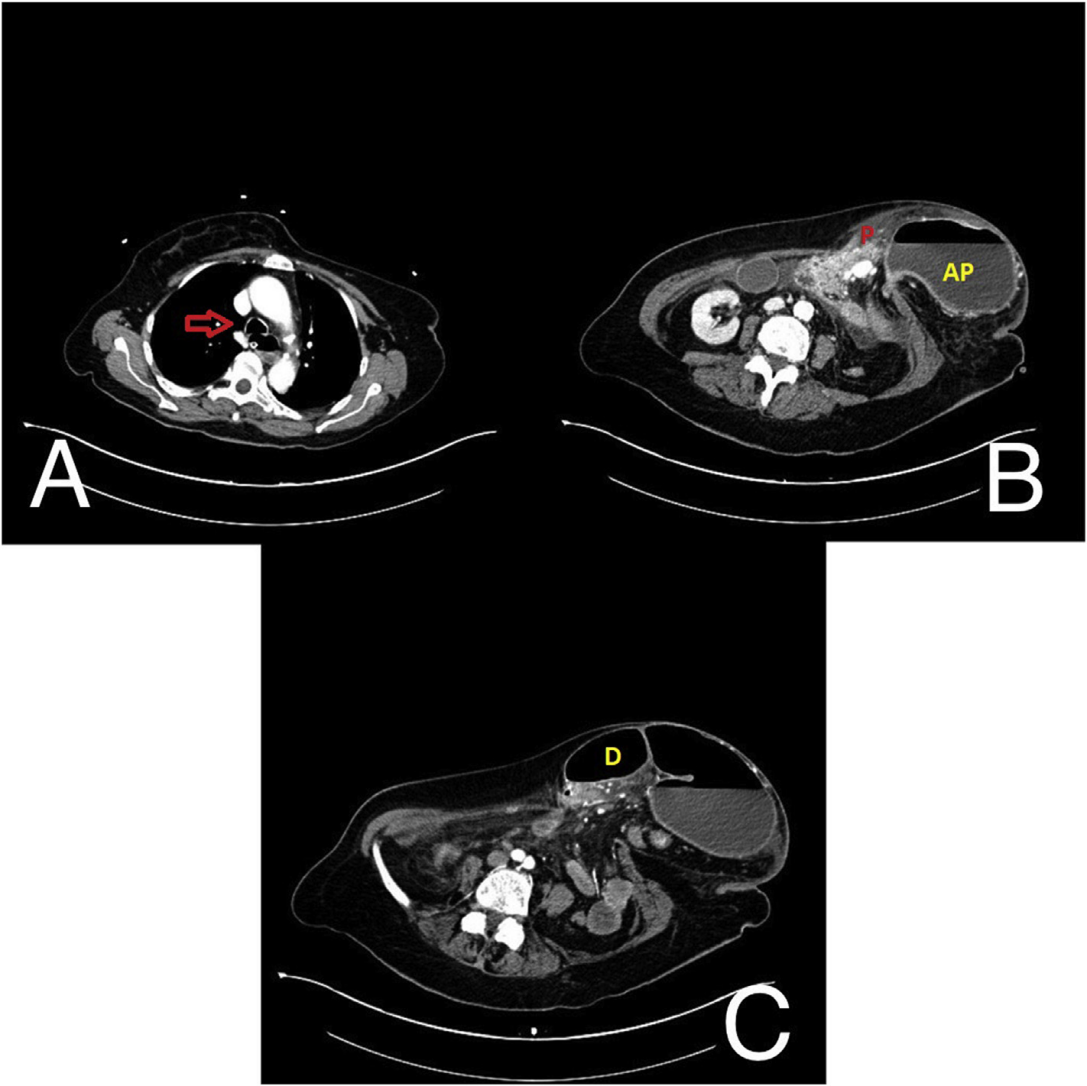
Pre-operative IV contrast-enhanced CT scan of the thorax and abdomen. Image A demonstrates the marked distension of the oesophageal lumen with air fluid levels (arrow). Image B demonstrates the voluminous paraumbilical hernia sac containing antro-pyloric portion of the stomach with air-fluid level (AP) and pancreas (P) protruding through an abdominal wall defect of about 6.5 cm. Image C demonstrates the markedly distended first portion of the duodenum (D) protruding into the hernia sac, pulling with it a portion of the pancreas.
